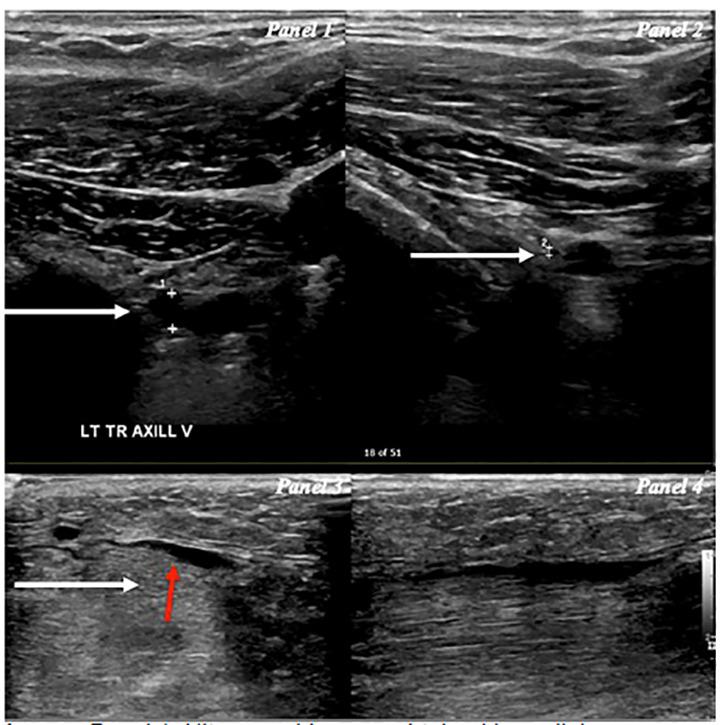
Panel 1, Ultrasound Images obtained by radiology technician: Left axillary vein in the transverse plane (white arrow), showing full compressibility (panel 2) with overlaying soft tissue swelling. Panel 3 with left upper arm with diffuse soft tissue swelling (white arrow) and interstitial edema (red arrow). Panel 4 areas of mixed echogenicity along with disorganized muscle fibers, consistent with findings of rhabdomyolysis.
